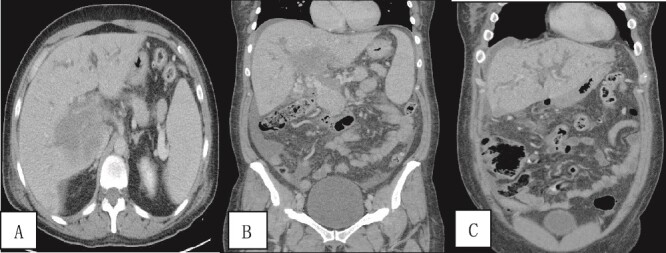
Contrast-enhanced CT scan of the abdomen and pelvis in (A) axial and (B, C) coronal sections obtained at the porto-venous phase showing a hypo-vascular geographic area with complex fluid density at the hilum of the liver with intrahepatic biliary dilatation. Non-opacification of the main portal vein with early cavernous transformation was noted as well as mild splenomegaly and ascites.
